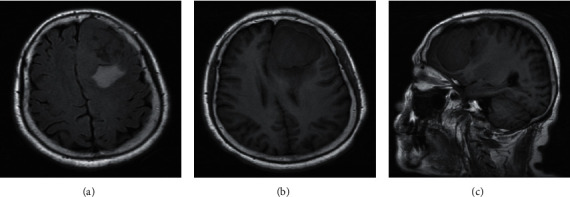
MRI image of a patient with psammomatous type of meningioma (male, 33 years old, left frontal tumor). (a) The patient shows equal and low confounding signals on axial T2WI, with clear boundaries, and no peripheral edema. (b) Axial T1WI shows basically uniform low signal. (c) Axial T1WI shows significant enhancement of tumor.
